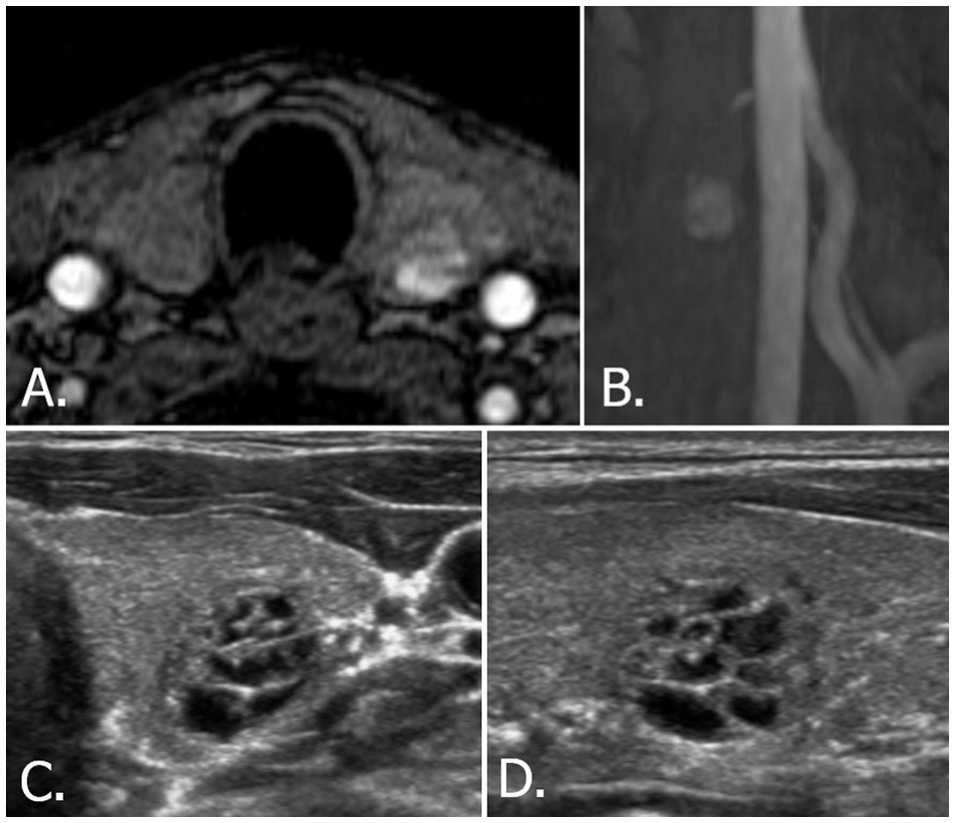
53-year-old male with left thyroid nodule. Source (A) and MIP (B) images of TOF-MR angiography show a totally hyperintense nodule in the left thyroid gland. Transverse (C) and longitudinal (D) US images of left thyroid reveals a spongiform nodule, which is classified as benign nodule (K-TIRADS category 2, ACR-TIRADS category 1, and EU-TIRADS category 2). MIP, maximum intensity projection; TOF-MR, time-of-flight magnetic resonance; US, ultrasound; K-TIRADS, Korean Thyroid Imaging Reporting and Data System; ACR-TIRADS, ACR Thyroid Imaging Reporting and Data System; EU-TIRADS, European Thyroid Imaging Reporting and Data System.
